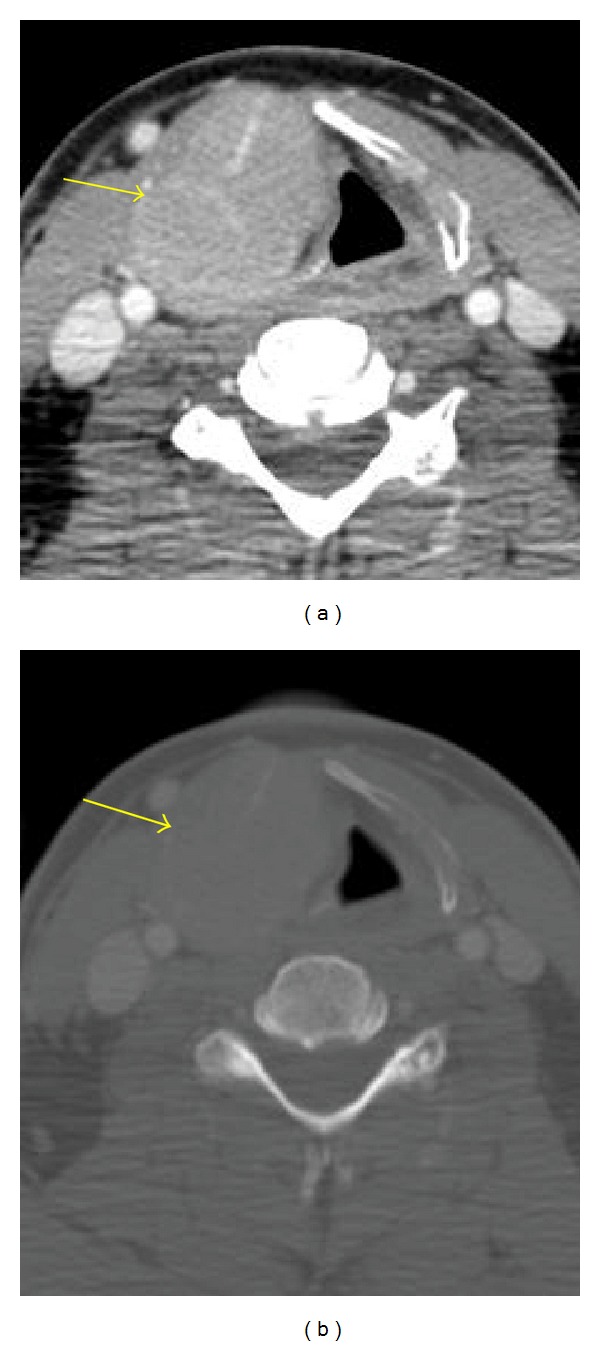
CT, postcontrast, soft tissue (a) and bone windows (b) Uniformly expanded right thyroid cartilage laminae.
